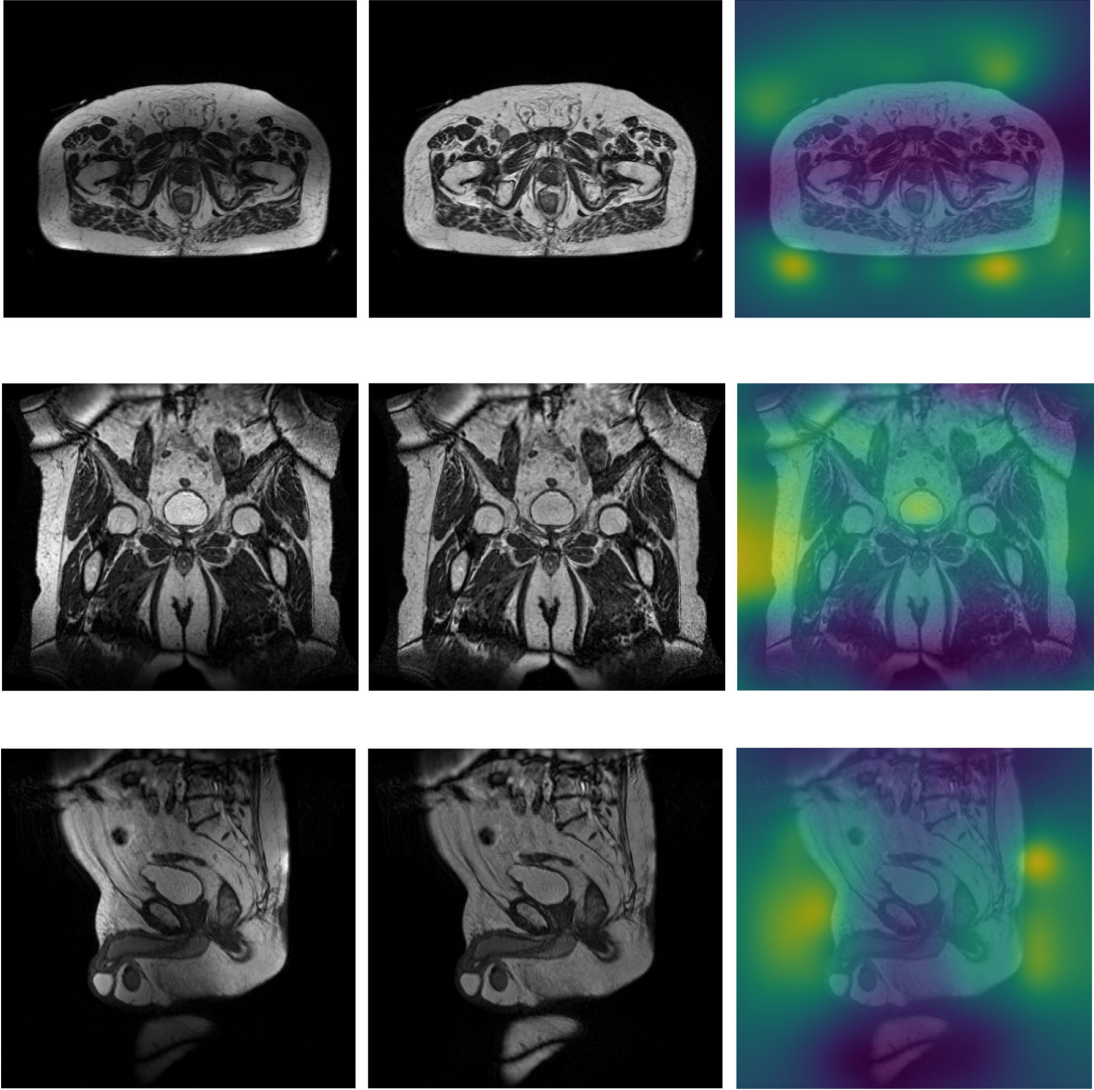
Axial (first row), coronal (central row), and sagittal (bottom row) central slice from the original MRI volume (left column), the bias-corrected MRI volume (central column), and the estimated bias field map with the chosen combination of N4ITK parameters (right columns), for a randomly selected patient. The darker region in bias field estimation (right column) indicates where the bias field is higher.
